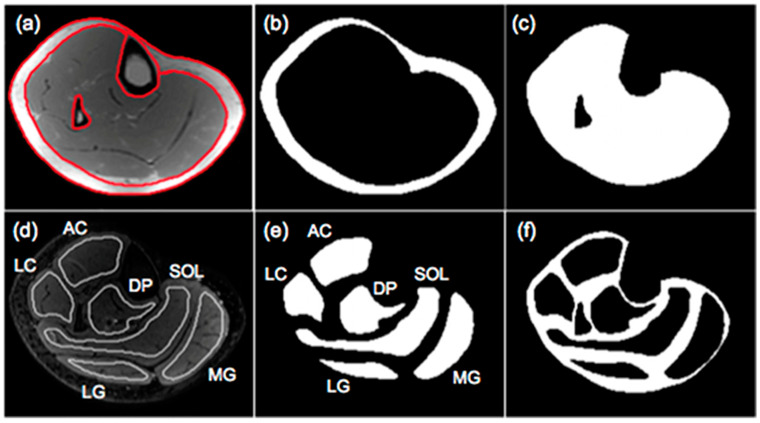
Segmentation of muscle and fat compartments: (a) typical in-phase IDEAL image and superimposed ROIs for subcutaneous fat and bone and bone marrow regions, (b) subcutaneous adipose tissue (SAT) mask, (c) mask including all the muscle regions and excluding the bone and bone marrow regions in the tibia and fibula, (d) typical T2-weighted FSE image and superimposed muscular ROIs used for the evaluation of fat distribution, (e) masks of 6 muscular ROIs, and (f) mask of soft tissue excluding subcutaneous fat and 6 muscular ROIs. Three muscles (medial gastrocnemius-MG, lateral gastrocnemius-LG, soleus-SOL) and three muscle compartments (anterior compartment-AC, lateral compartment-LC, deep posterior compartment-DP) were used to define muscular regions. Fat within the mask of (c) corresponds to IMAT, fat within the mask of (e) corresponds to intraMF, and the fat within the mask of (f) corresponds to interMF. Reproduced with permission from the authors in Ref. [43].
